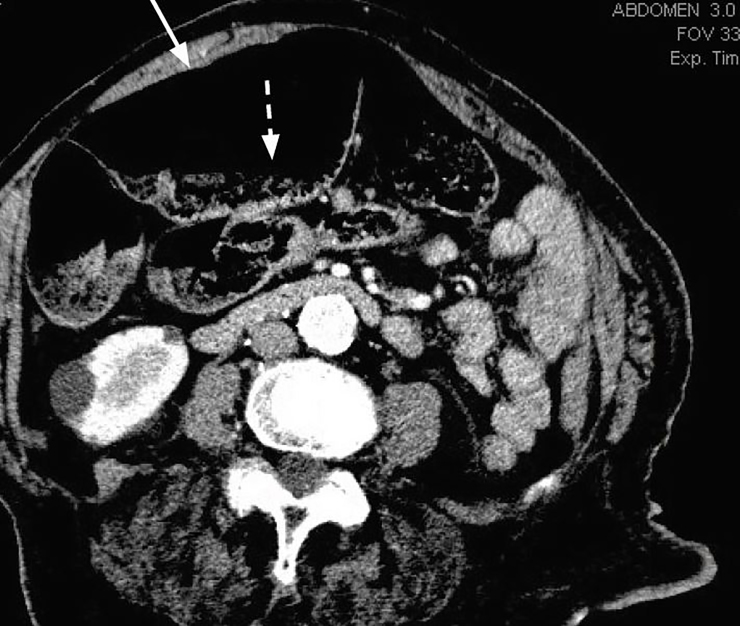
Computed tomography axial image demonstrating gaseous distension of the cecum (solid white arrow) with visualized air-fluid levels within the bowel (dashed white arrow).
